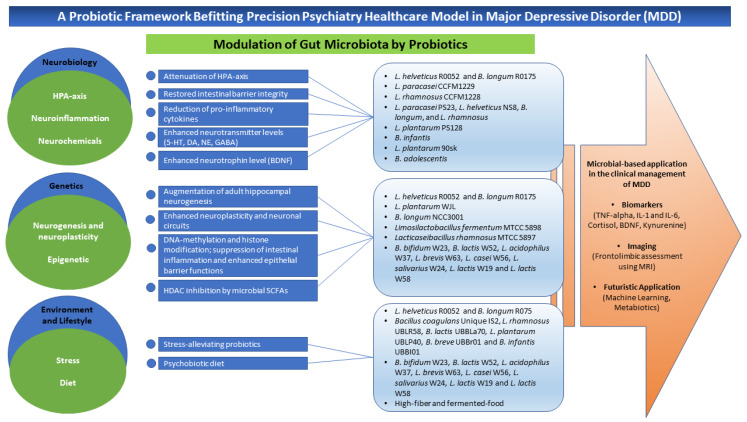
A probiotic framework befitting the precision psychiatry healthcare model in MDD. This framework represents three main components of precision psychiatry: neurobiological bases, genetic bases, and environment and lifestyle elements of MDD that involve microbial intertwining. Modulation of gut microbiota is the rudimentary anti-depressive mechanism of probiotics. Some commonly explored probiotics of *Lactobacillus* spp. and *Bifidobacterium* spp., either as a single strain or multi-strain, exert anti-depressive mechanisms by acting on different pathophysiologic mechanisms implicated in depressive disorder. Stress and diet are mainly modifiable targets in the prevention of MDD. The microbial-based applications in the clinical management of MDD include some potential biomarkers obtainable through a patient’s blood analysis, assessment of vital frontolimbic regions in the brain using MRI, and integration of machine learning and development of depression-specific metabiotic as part of futuristic vision within this context.
